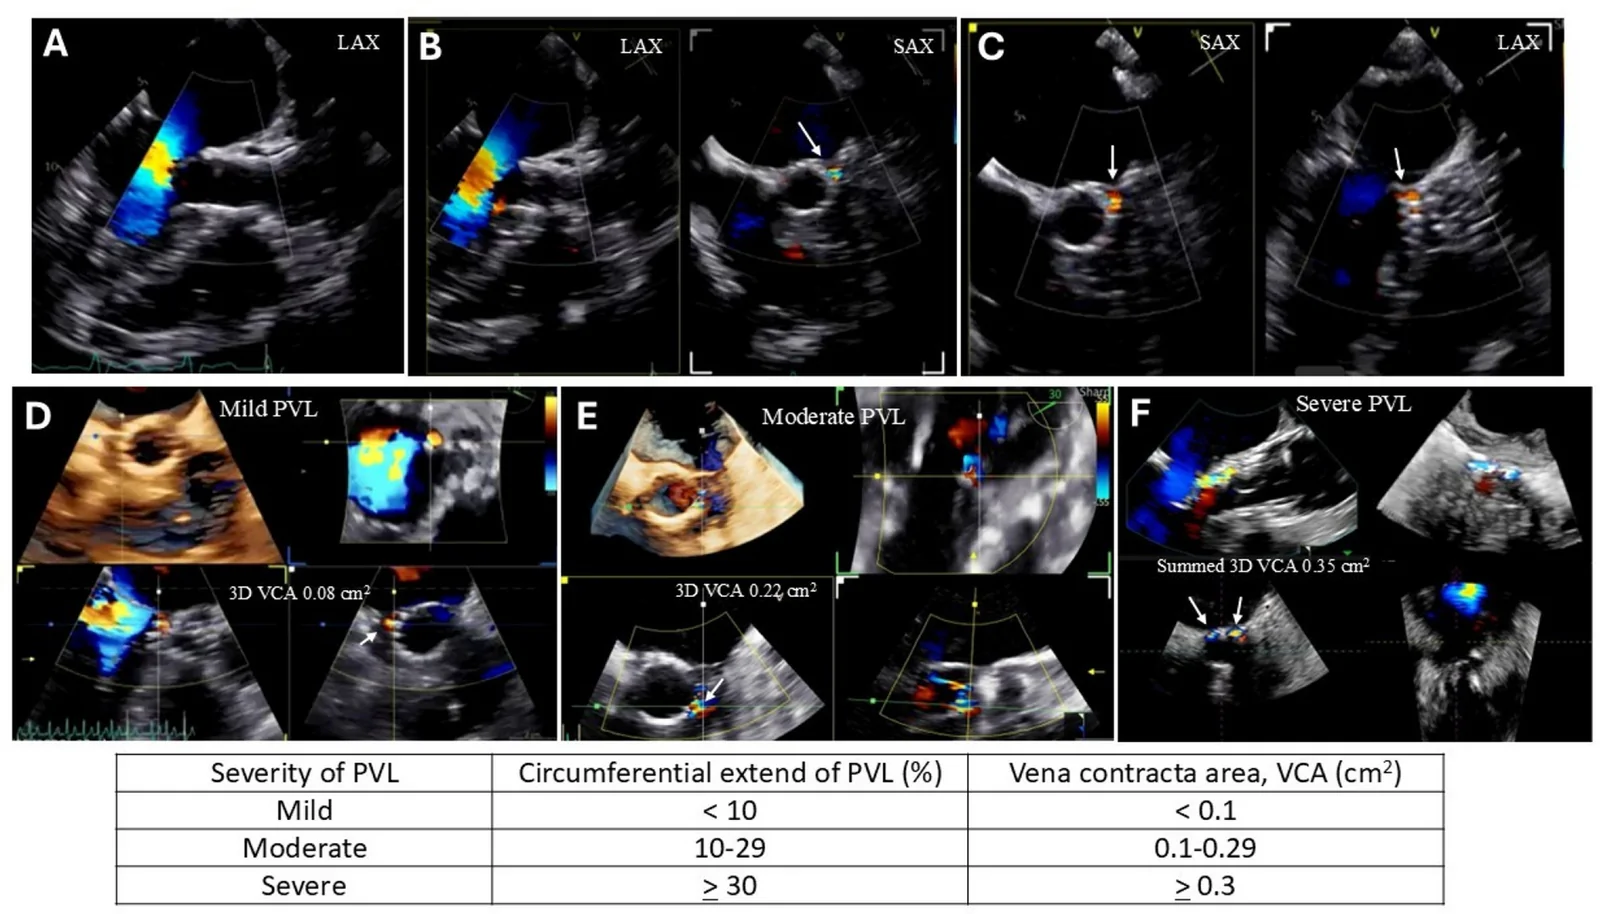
Assessment of paravalvular aortic regurgitation on transesophageal echocardiography. A Initial mid-esophageal long-axis (LAX) view showing no paravalvular leak (PVL), simultaneous biplane imaging with cut-plane at the level of inflow edge of the transcatheter valve to locate the PVL, at 2 o’clock of B the corresponding short-axis (SAX) view. C Further rotation of the probe confirming the eccentric PVL jet, in the off-axis mid-esophageal LAX view. Severity of PVL is graded by its circumferential extent: less than 10% is considered mild, 10% to 29% is moderate, and greater than 30% is severe; or based on the planimetered 3D vena contracta area (VCA) of the PVL jets. (D–F) For multiple PVL jets, summation of all their respective 3D VCA may be employed
